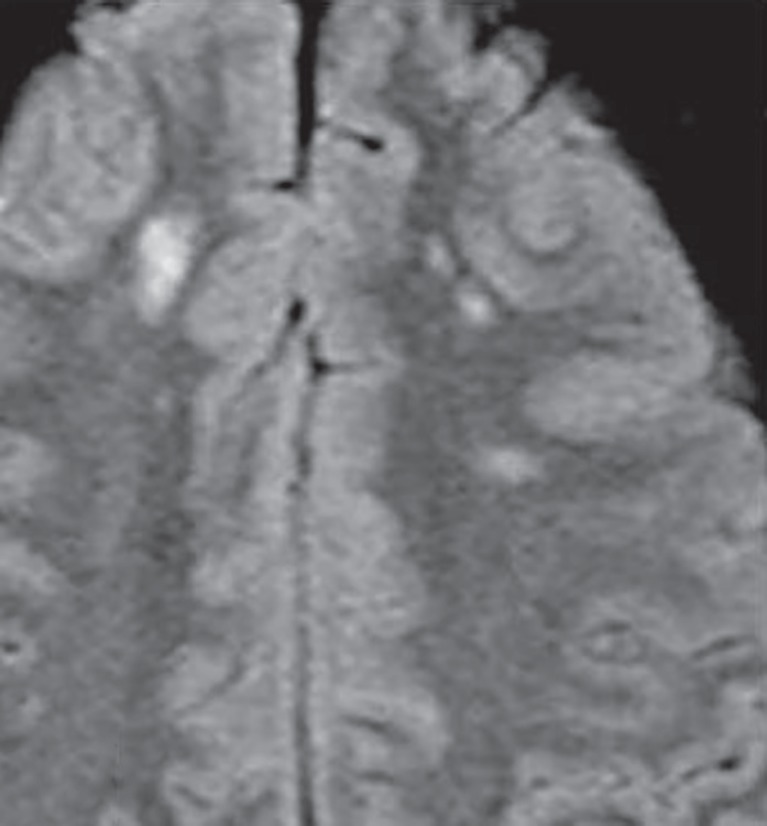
Diffuse axonal injury (DAI). Punctate FLAIR hyperintense foci in subcortical and deep white matter which are difficult to pick up on CT scan images in post trauma setting
